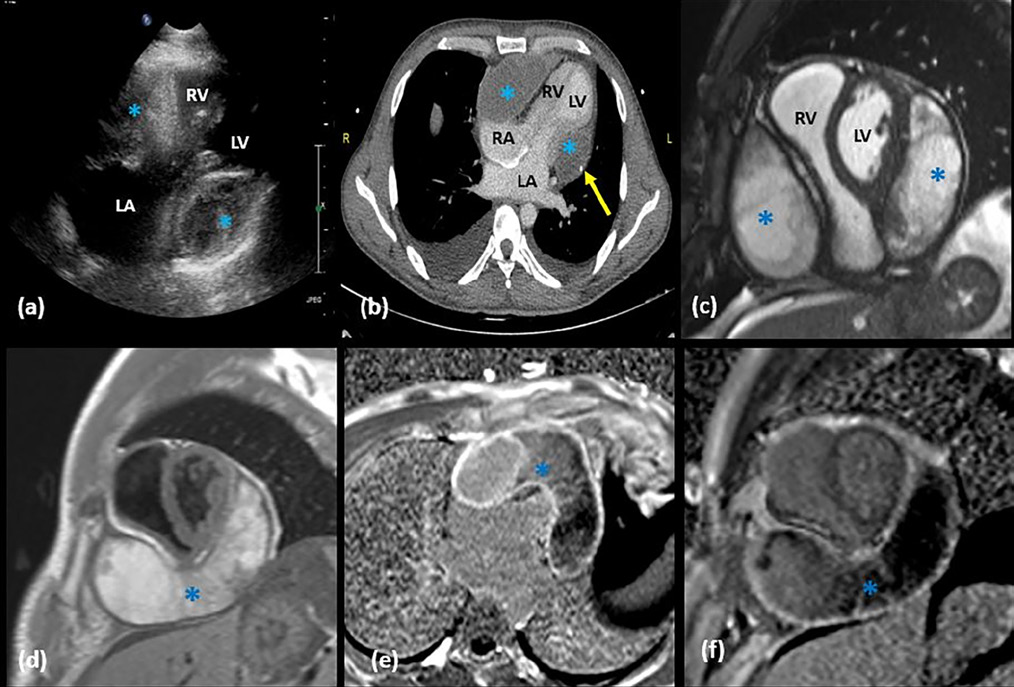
1(a) Echocardiogram (in IU 22, Philips) done in apical 4 CH chamber view shows a heterogeneous hyperechoic lesion (*) in the right and left AV groove region causing significant compression on the LV & RV chambers. 1 (b) Post contrast CT [in Brilliance ICT 256 slice, Philips Healthcare, Cleveland, OH] axial section showing hypodense cystic lesion (*) along the right and left AV groove with peripheral wall enhancement. A focus of calcification (yellow arrow) is noted in the thick wall towards the left side. In addition, bilateral pleural effusion secondary to heart failure is also seen. 1 (c–f) Cardiac MRI [in Avanto Fit 1.5T,Siemens, Erlangen, Germany)], SA images (c, d, f) and 4 CH image (e) showing the predominantly hyperintense thick walled cystic lesion (*) with horseshoe configuration (d–f) along the AV groove posteroinferior to the heart. T1 SA slice (d) showed hyperintense content indicative of haemorrhagic content. Post-contrast delayed PSIR image (e, f) showing the peripherally enhancing thick wall of the cyst. Otherwise, no significant pericardial effusion was detected in the present imaging. AV, atrioventricular; 4 CH, four chamber; LA, left atrium; LV, left ventricle; PSIR, phase sensitive inversion recovery; RA, right atrium; RV, right ventricle; SA, short axis.
